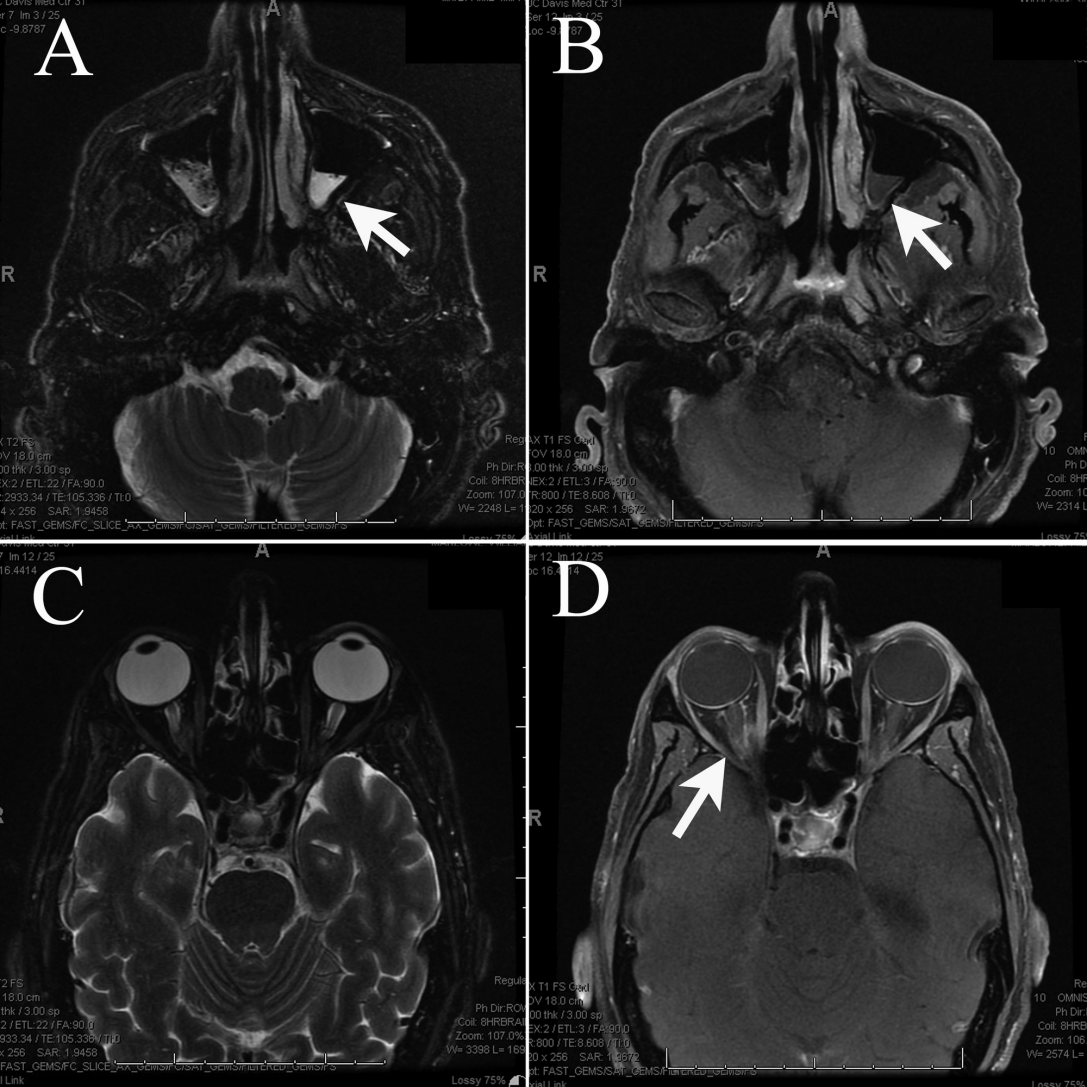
Magnetic resonance imaging (MRI) of the orbit/face/neck A) Axial T2 MRI and B) Axial T1 post-contrast MRI showing mild ethmoidal and maxillary sinus mucosal disease with fluid/air levels, *white arrows* indicate fluid/air levels in maxillary sinus. C) Axial T2 MRI and D) Axial T1 post-contrast MRI showing bilateral optic neuritis with suggestion of a more acute process on the right, *white arrow* indicates contrast enhancement on right optic nerve.
